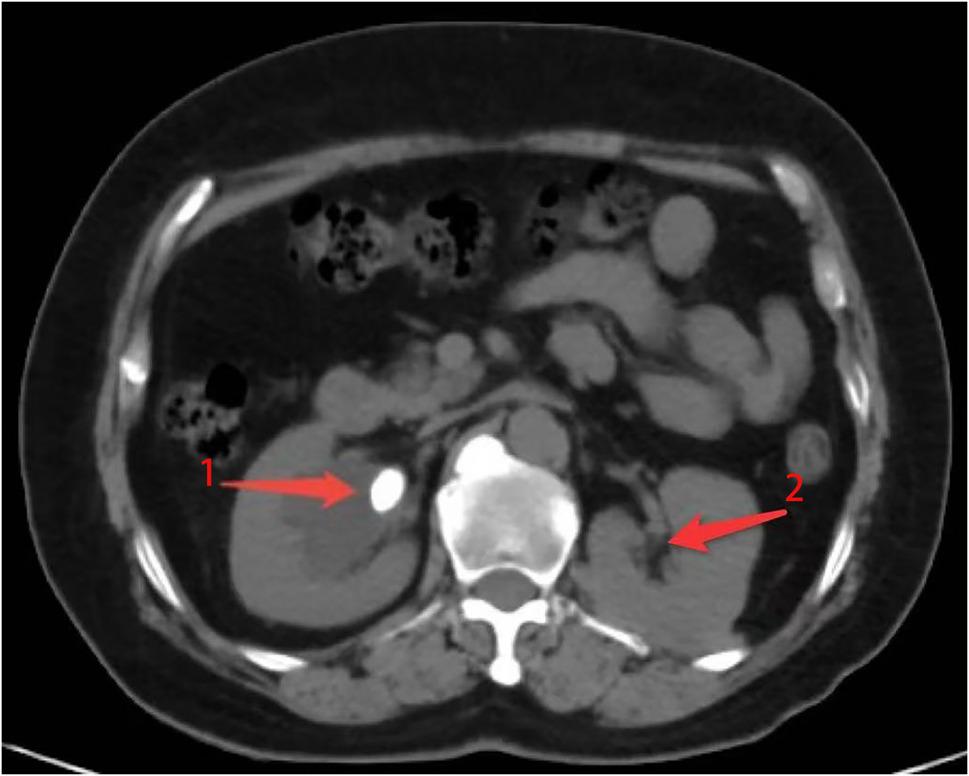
Abdominal CT scan showing findings in the right and left kidneys. Arrow 1 indicates a hyperdense right renal calculus with mild hydronephrosis, measuring approximately 2.1 × 1.4 cm. Arrow 2 points to mild, atypical hypodense lesions in the left kidney
